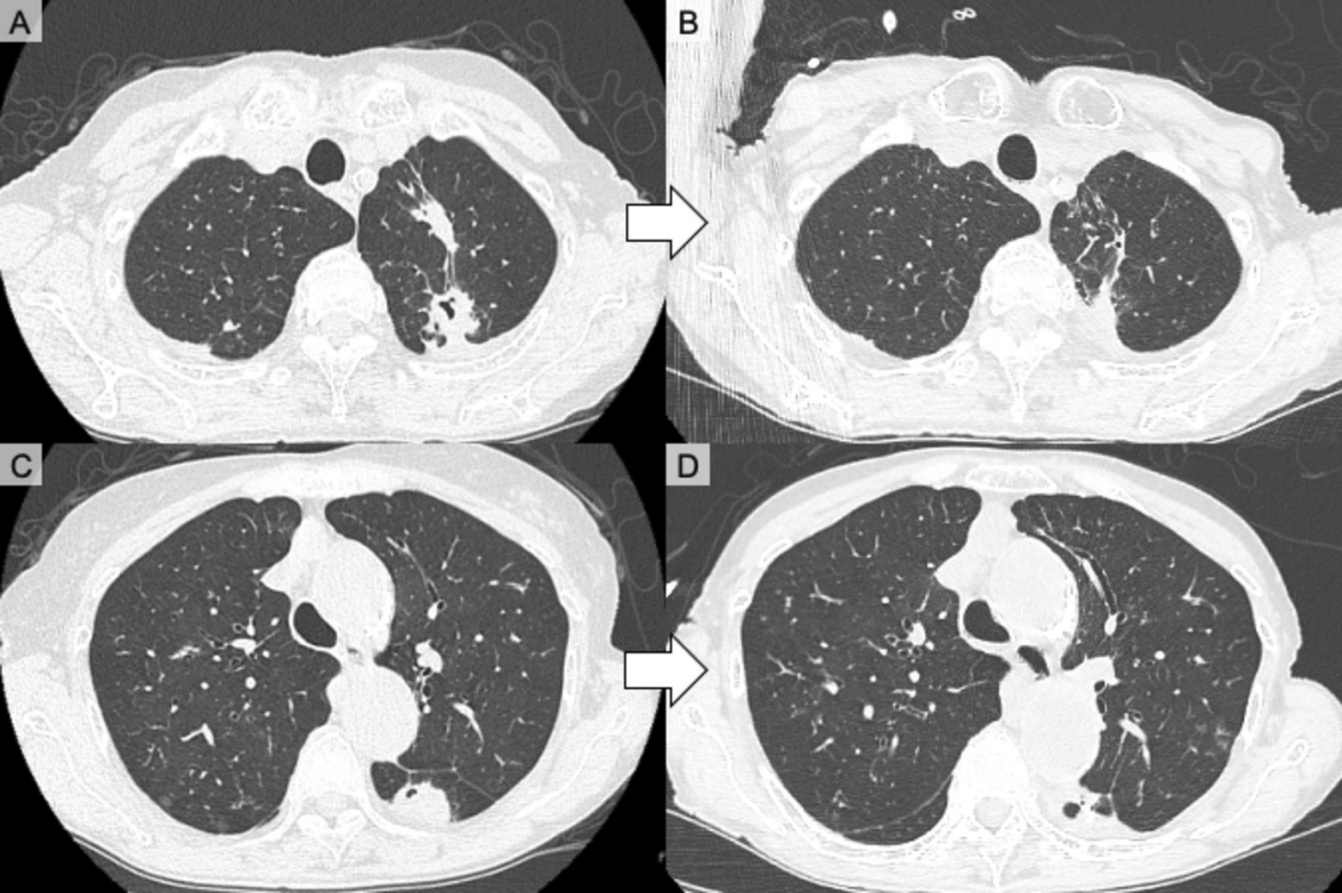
Chest computed tomography (CT) findings before and after treatment with infliximab. (A, C) CT images showing multiple nodular infiltrates in both lungs with occasional cavitation before treatment. (B, D) CT images of the same slice levels demonstrating marked improvement including reduction of nodular infiltrates and resolution of cavitary changes after treatment.
